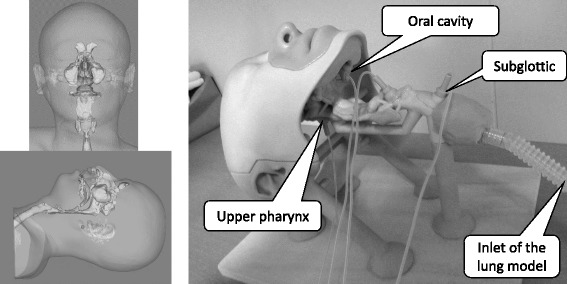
The airway model. The actual airway model was made by a 3D printer using 3D-CT data. Sampling ports were made to record capnograms at each site in the model
